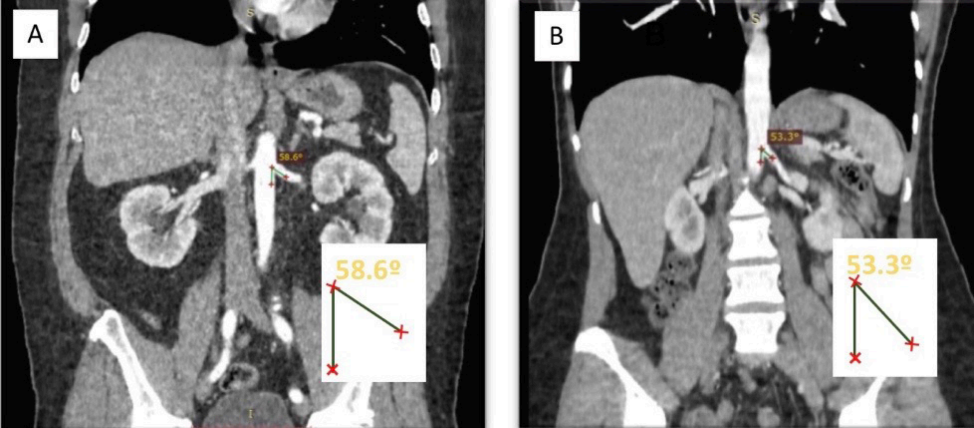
Abdominal computed tomography with contrast in coronal view, comparing measurements of the angle of origin of the left renal artery (LRA) in patients of both sexes aged 61 to 70 years. (A) angle of origin of the LRA in a man; (B) angle of origin of the LRA in a woman.
